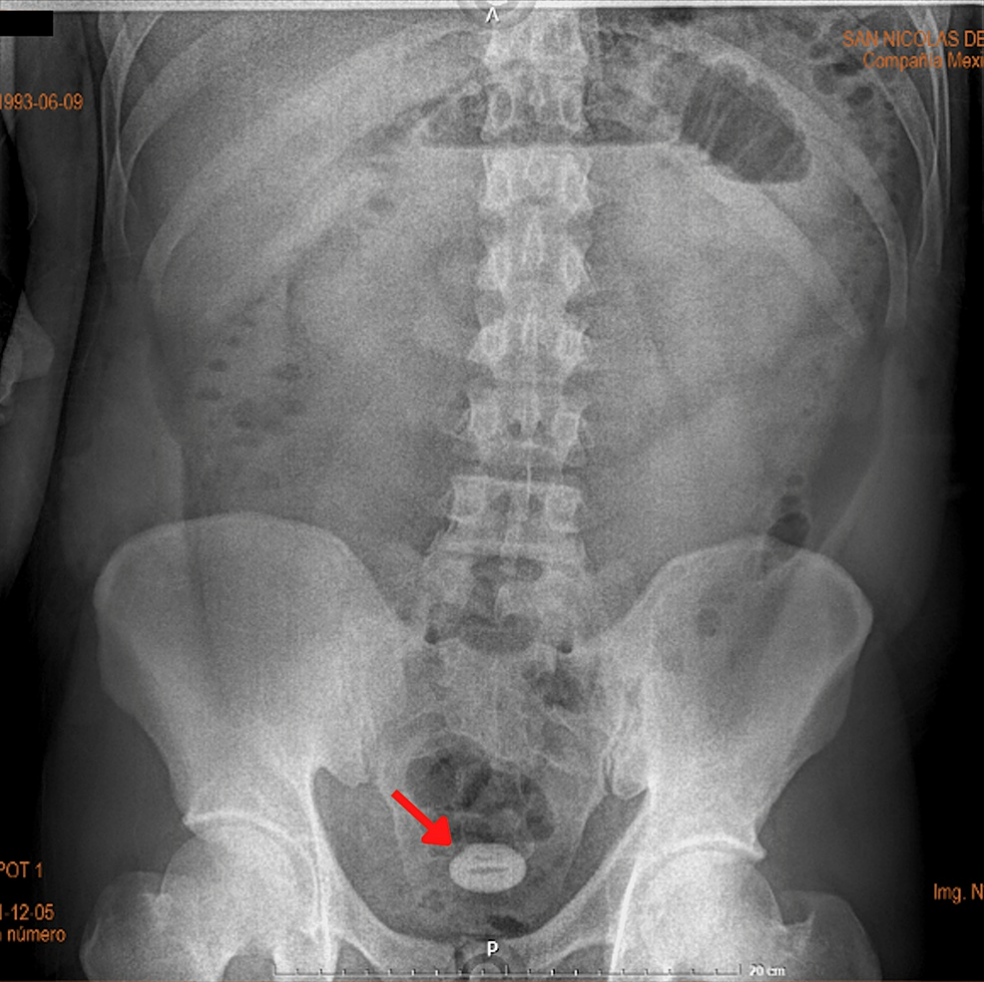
Abdominal X-ray showing the gallstone (the red arrow).
